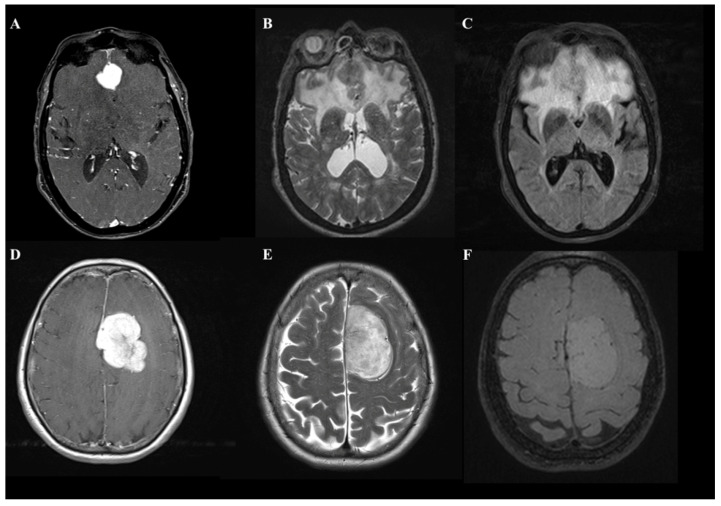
A comparison of two cases of patients with frontal lobe meningioma with an asymptomatic onset that, on MRI images, document entirely different volumes of edema. In the first case, T1-weighted with MDC (A), T2-weighted (B), and FLAIR (C) images show a 2 cm para-alpine lesion with edema involving the entire frontal lobe. In the second case, the identical sequences (D–F) show a more than 4.5 cm lesion in diameter with a volume of edema almost absent.
